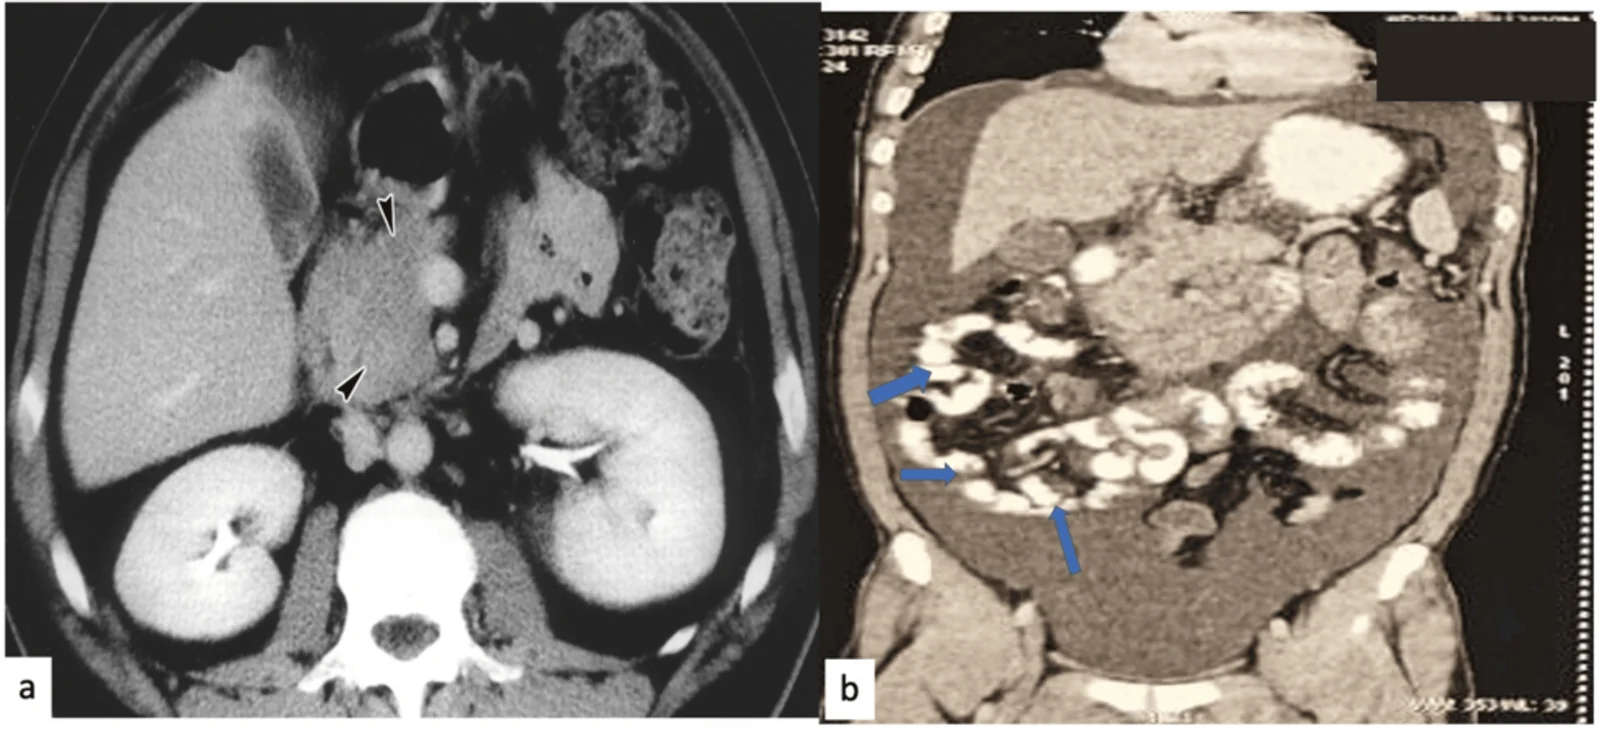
Contrast-enhanced computed tomography (CECT) of the abdomen. (a) CECT of the abdomen revealing a retroperitoneal mass lesion (black arrowhead). (b) CECT of the abdomen showing multiple mural wall thickenings in the small intestine leading to stricture and luminal narrowing (blue arrows).
